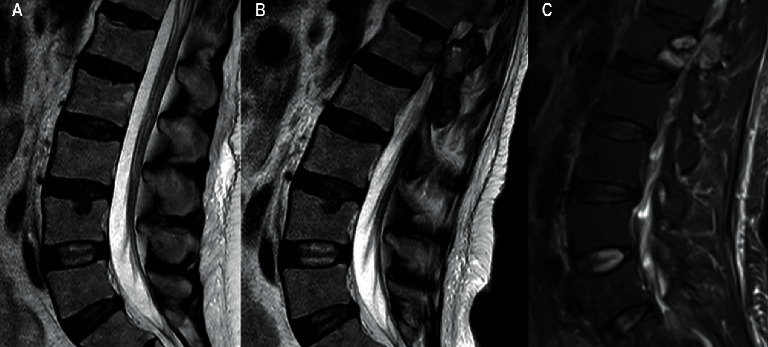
Sagittal spine MRI. (a) T2 weighted sequence from the same investigation as the latest thoracic image showing no lesions in L1. (b) T2 weighted sequence one year later showing tumor in the body and pedicle of L1. (c) T2 weighted Dixon fat suppressed sequence 9 months later showing no further progression of the tumor.
